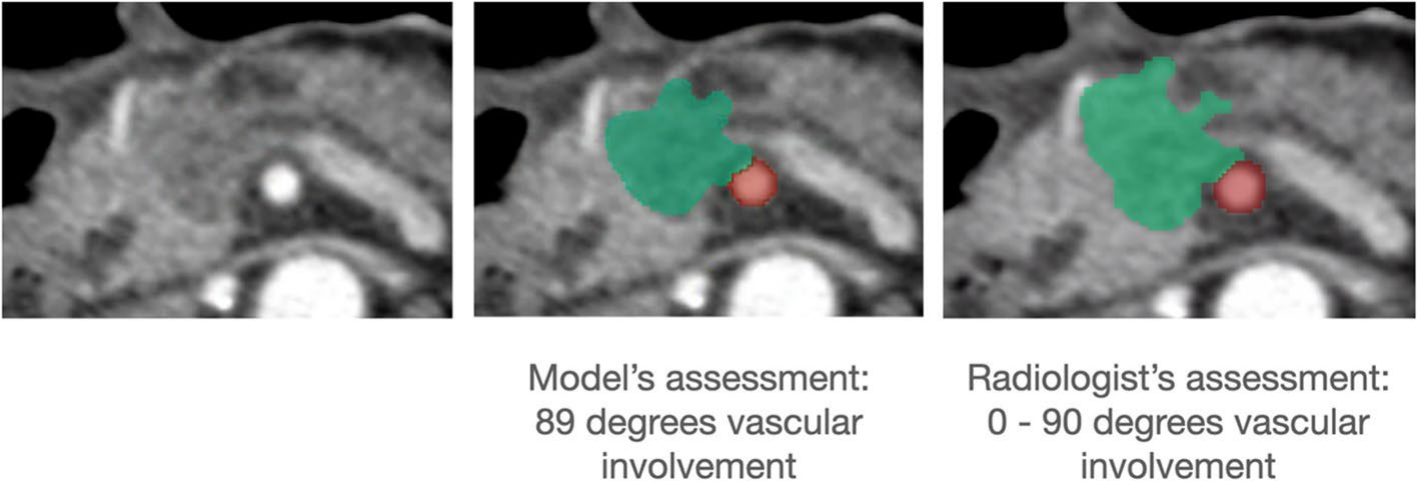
Example of a manually and an artificial intelligence (AI)-segmented computed tomography late arterial phase scan with the PDAC and superior mesenteric artery. Green = PDAC tumor; red = superior mesenteric artery. Using the AI-generated segmentation, our model quantifies the vascular involvement of the superior mesenteric artery by the PDAC tumor at 89°, which is in line with the radiologist’s assessment (0–90°). *PDAC*, Pancreatic ductal adenocarcinoma
